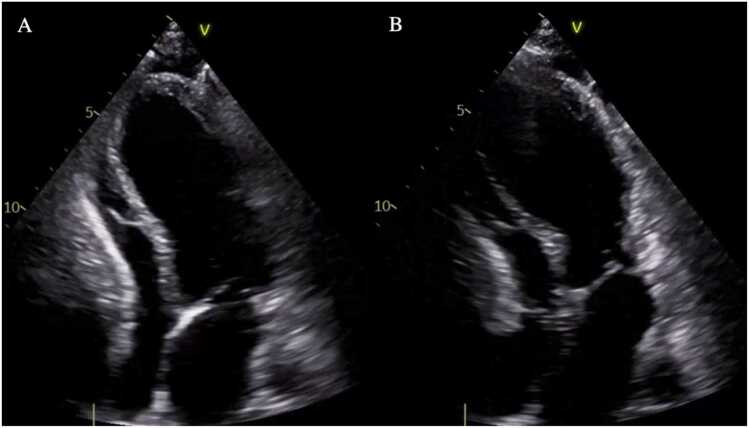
Transthoracic echocardiogram, 2-chamber view of patient 2 showing typical Takotsubo findings. (A) End-diastolic image. (B) End-systolic image showing akinesia of the mid and apical segments.
